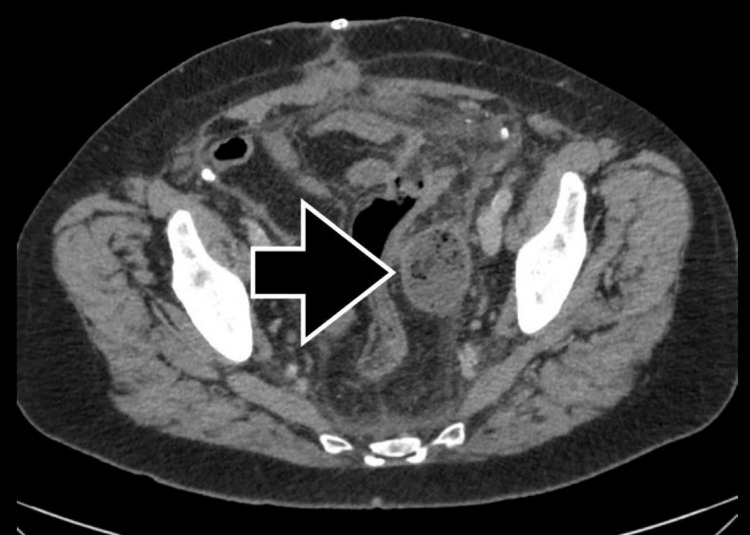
CT image from postoperative day 11 demonstrating an air-containing fluid collection measuring 3.6 × 4.6 × 4.8 cm in the left lateral pelvis. CT: computed tomography
